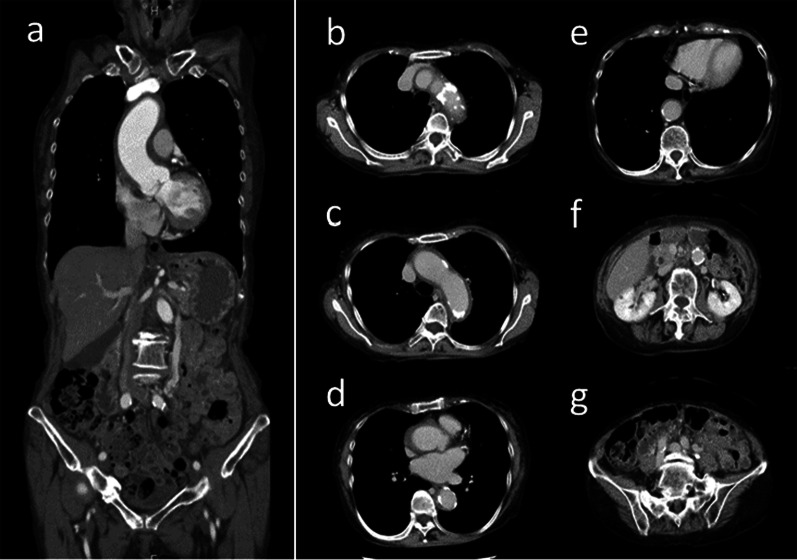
Computed tomography imaging of acute type A aortic dissection. a Dissected ascending aorta. The maximum short diameter of the ascending aorta was 39 mm, and the maximum thickness of the thrombosed false lumen was 4 mm. b, c Dissected aortic arch. d Dissected aortic root and descending aorta of left atrial level. e Dissected descending aorta. f Non-dissected infrarenal aorta. g Non-dissected iliac arteries
